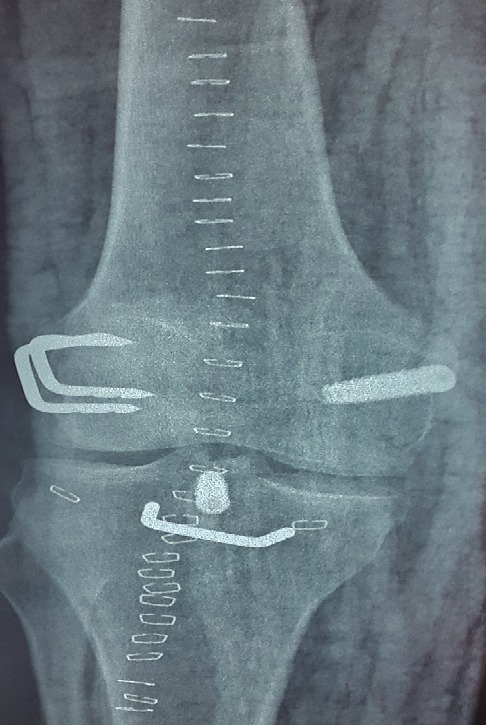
Radiographie post-opératoire du genou droit
